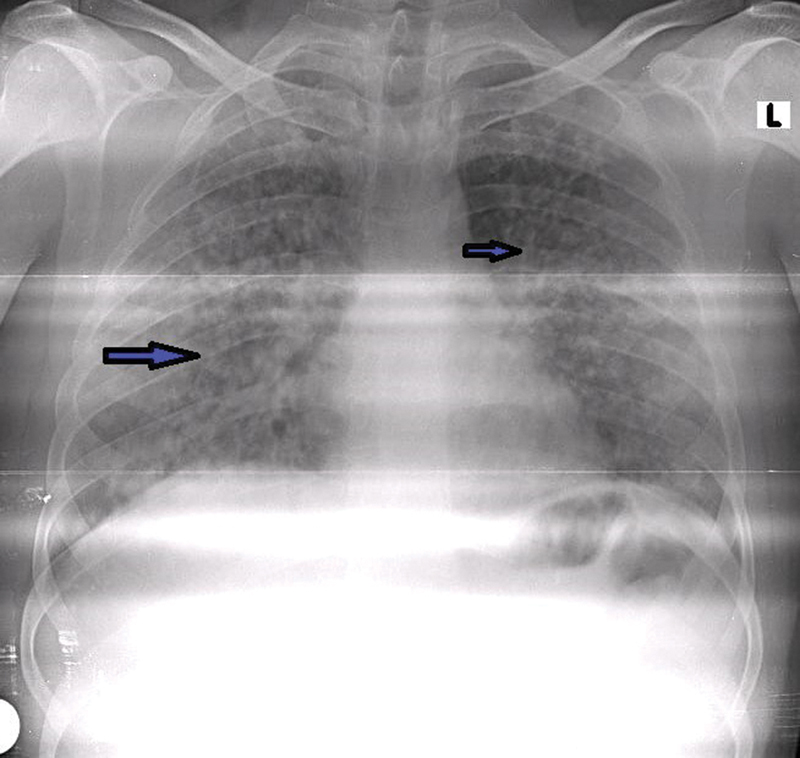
Chest X-ray showed diffuse nodular infiltration in all lobes. Arrows highlight regions of diffuse nodular infiltration in all lung lobes on the chest X-ray.
